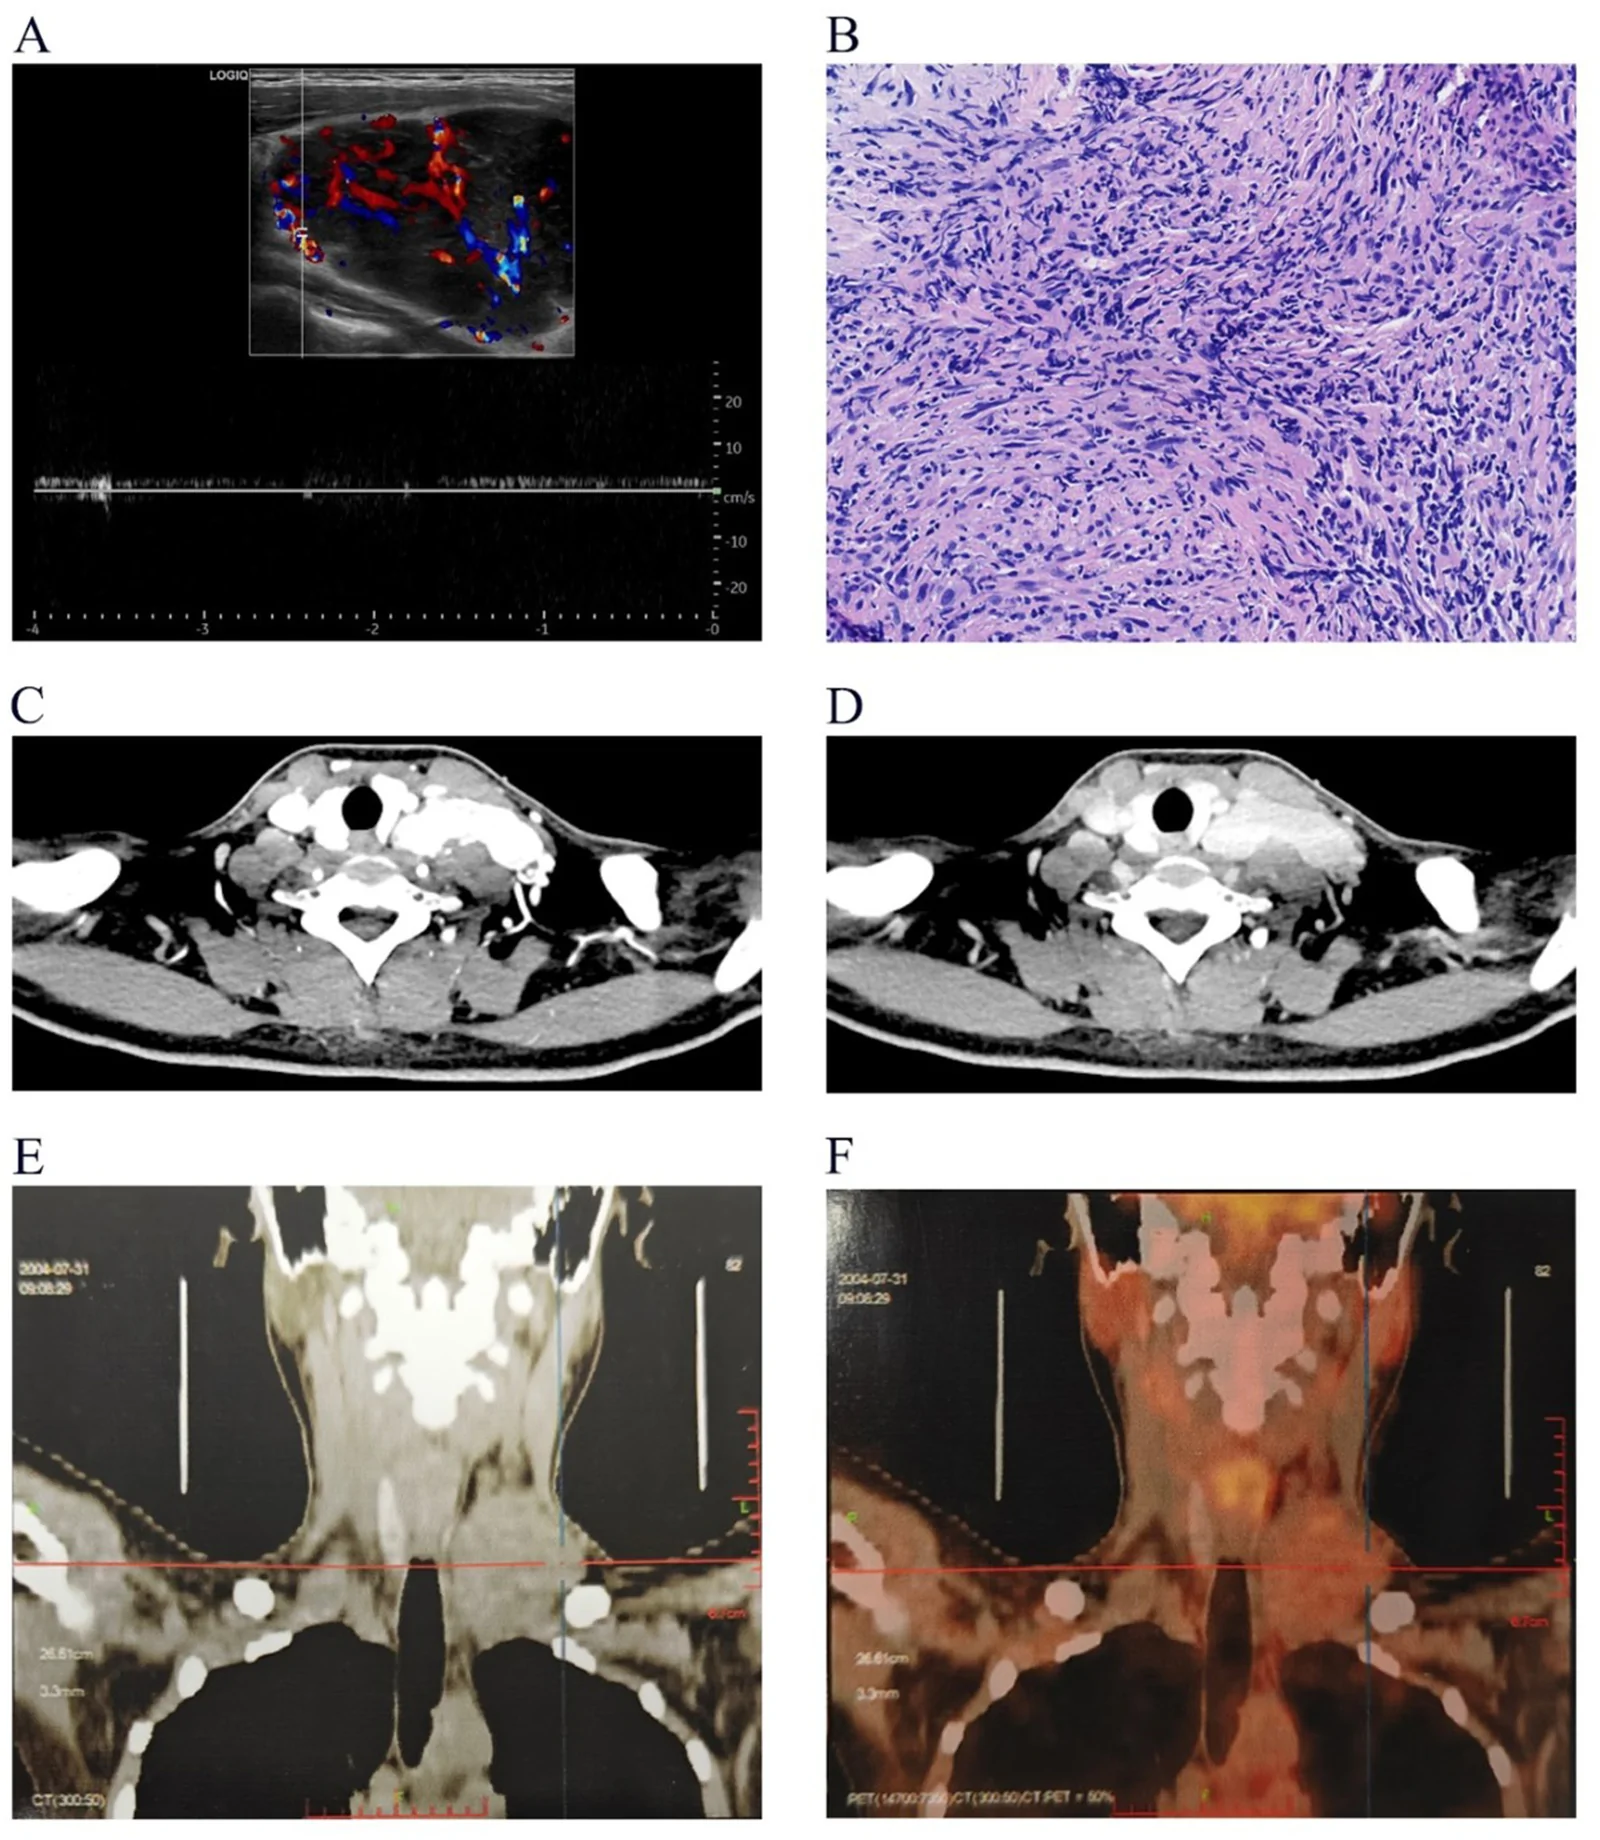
Preoperative imaging and core-biopsy findings. (A) Ultrasonography shows a well-circumscribed hypoechoic lesion with abundant internal vascularity. (B) Core biopsy shows spindle-cell proliferation. (C, D) Contrast-enhanced CT shows marked enhancement and internal vascular structures. (E, F) PET/CT shows heterogeneous FDG uptake (SUVmax, 1.9).
